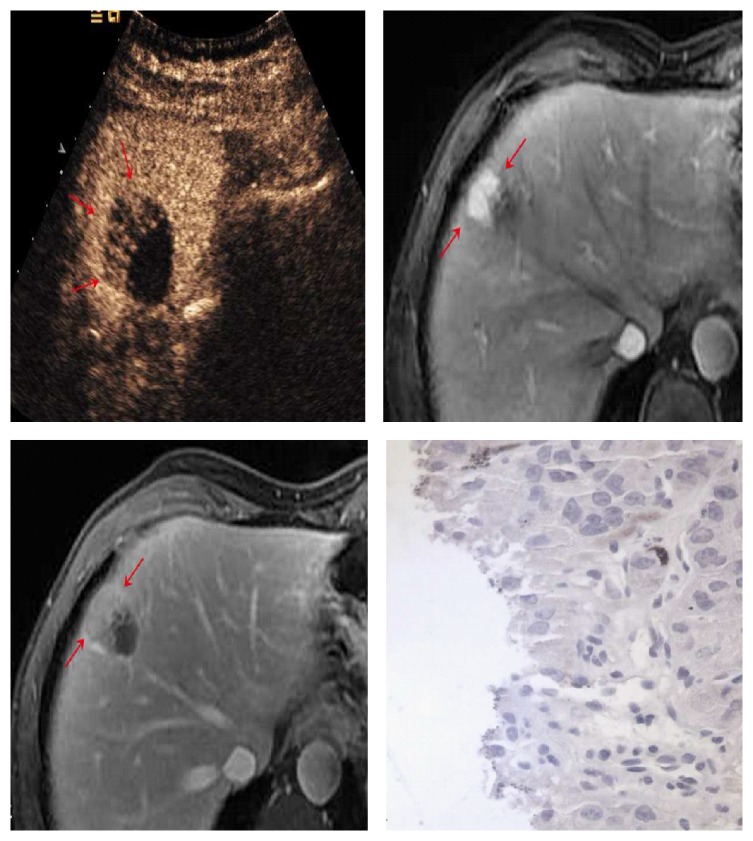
40-year-old man with 3.3 cm×3.2 cm HCC nodule. In portal venous phase, the portal venous phase, the partial enhancement seen at CEUS washed out gradually (a) (red arrows). MR images showed arterial phase enhancement with progressive washout in late phase imaging (b, c) (red arrows). Pathological results showed residual hepatocytes (d).
